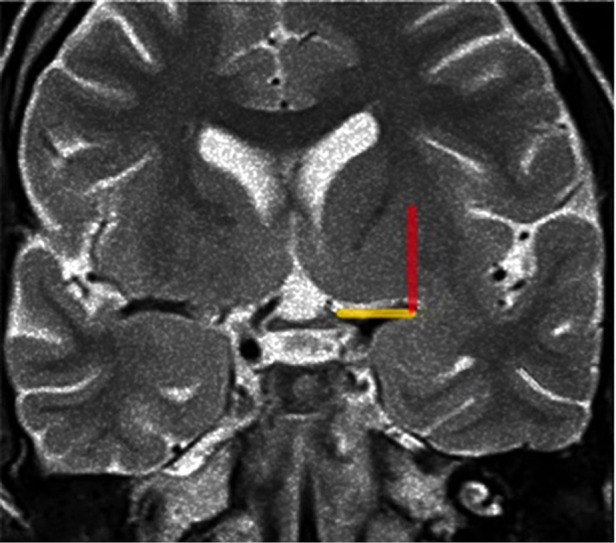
Coronal T2-weighted MRI at the level of the optic chiasm as an imaging parameter of the anterior perforated substance. The imaginary line (the Optic Chiasm – Insular Recess line) is drawn, from the optic chiasm medially to the insular recess laterally, to identify the anterior perforated substance (yellow line). The Porto Alegre line (Red Line) is as ascendent line from the lateral end of the OC-IR line and represents the lateral limit of the lateral LSTa inside the central core. In cases which the medial border of insular tumor crosses this line medially there is a great probability of involvement of the LSTa by the tumor.
